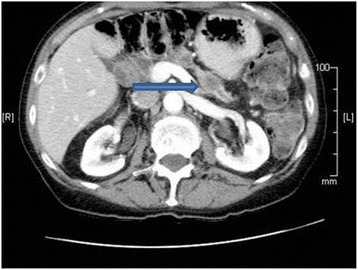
Representative cut of the patient’s CT scan in March of 2013, 4 years after the initial surgery. This demonstrates a mass within the pancreatic remnant suspicious for carcinoma
